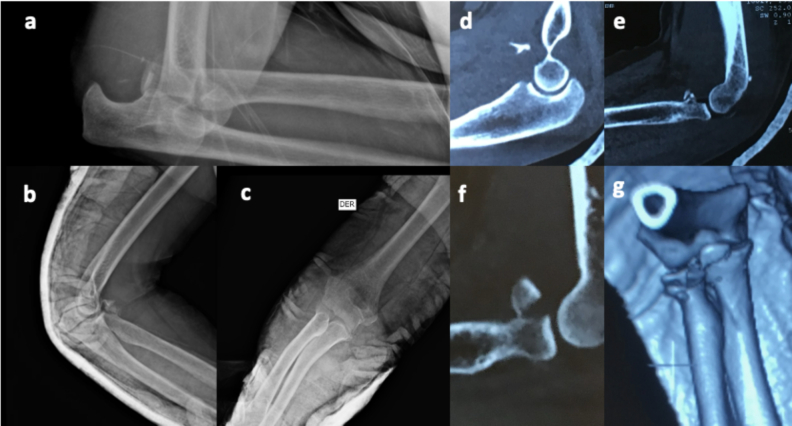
a) Lateral radiograph of the right elbow showing posterior elbow dislocation; b,c) lateral and AP radiographs respectively demonstrating reduction of dislocation + coronoid and radial head fractures; d,e,f) Sagittal cuts showing coronoid and radial head fractures; g) 3D reconstruction.
